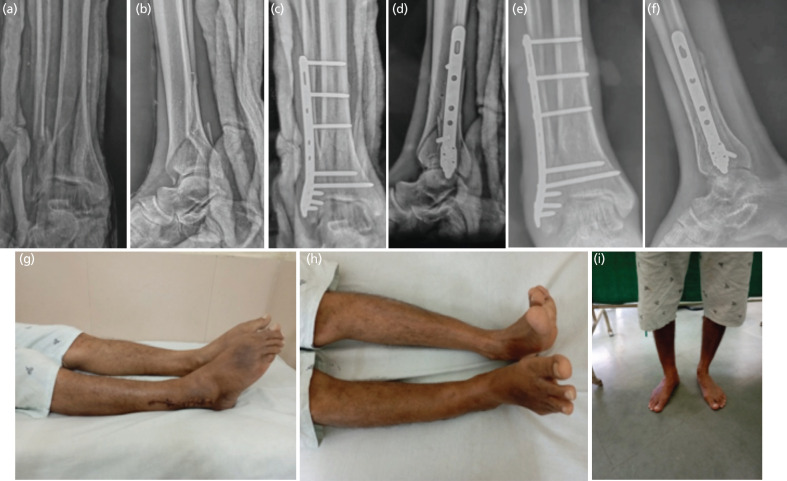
(a, b) AP and lateral pre-operative, (c, d) immediate, and (e, f) six-month follow-up radiographs. (g, h, i) Clinical pictures of a 26-year-old male with right side distal tibial fracture managed with fibula pro tibia plating, showing good function, alignment, and union.
